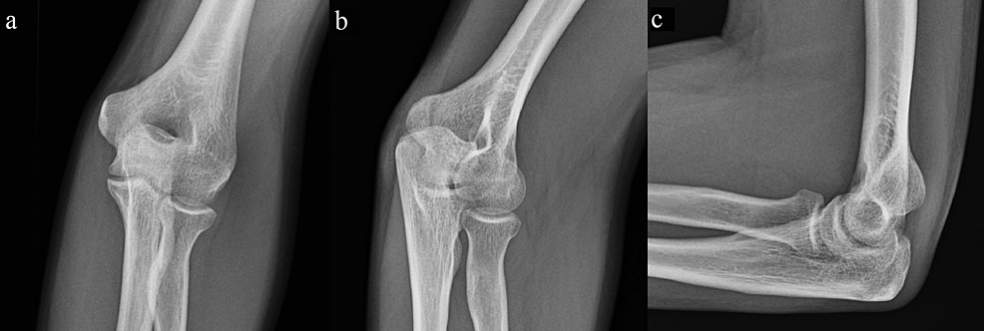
Preoperative radiographs of the left elbow Anteroposterior (a), oblique (b), and lateral (c) radiographs of the left elbow demonstrate no visible abnormality.
